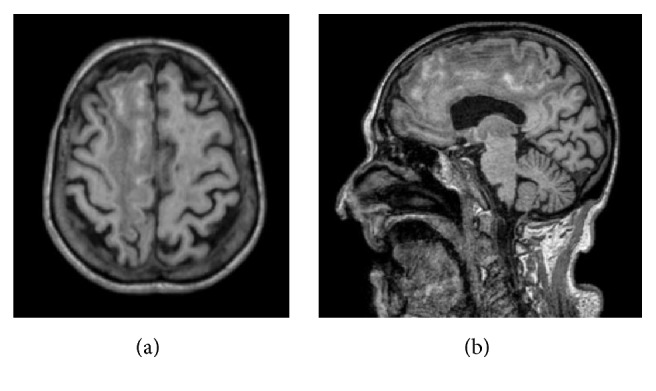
Axial (a) and sagittal (b) T1 weighted MR images. Linear spontaneous hyperintensity of the cortex in the frontoparietal convexity, compatible with laminar necrosis.
